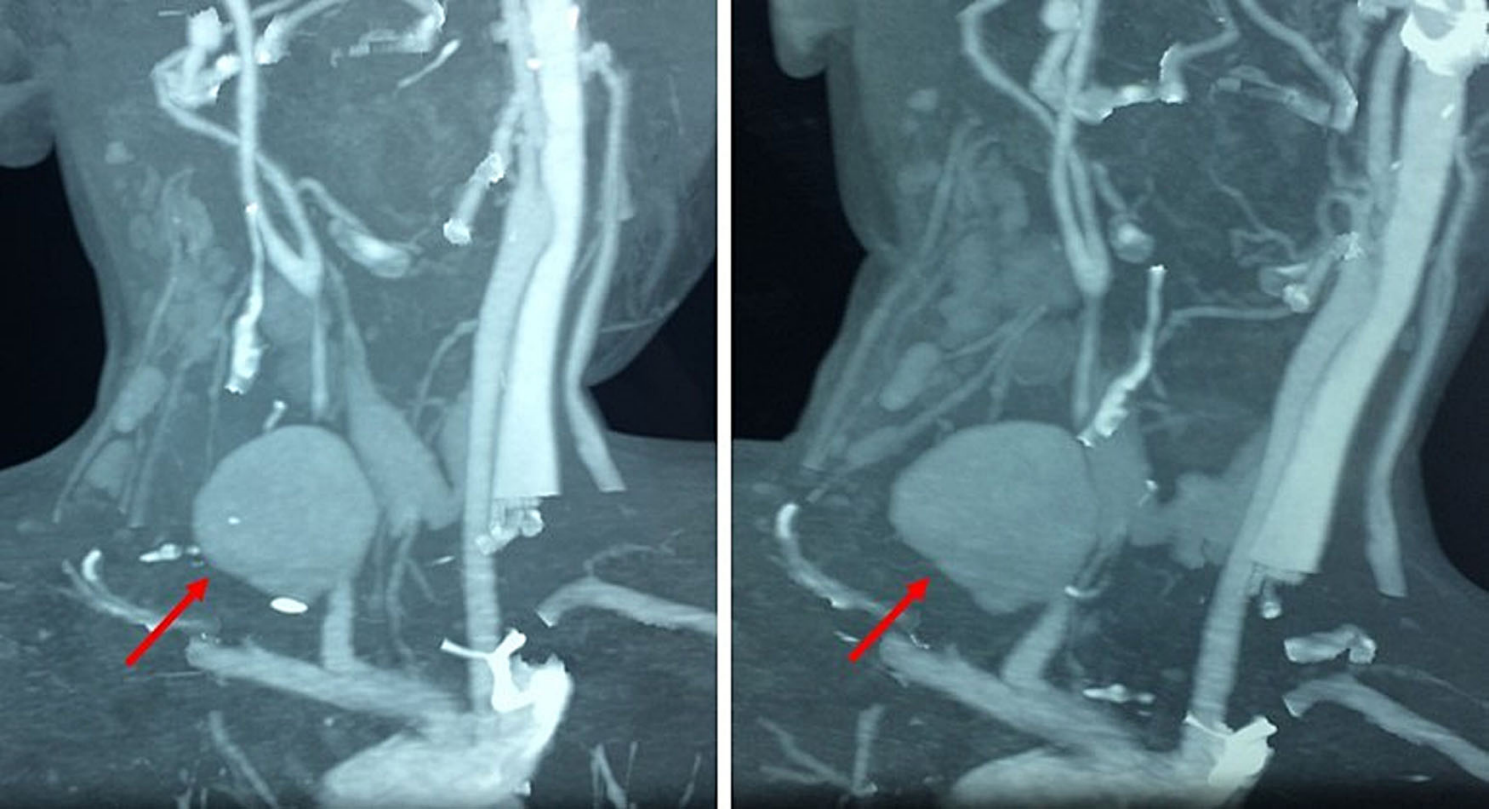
Cervical computed tomography angiogram showing a 3 cm right common carotid artery pseudoaneurysm beginning at 2 cm of its origin and at a distance from the carotid bifurcation (red arrow), with the presence of multiple cervical lymphadenopathies.
